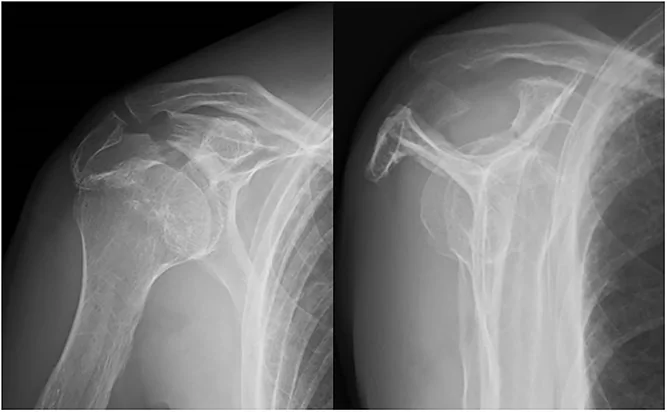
Preoperative radiographs of the right scapula showing displaced acromion and coracoid process fractures.
